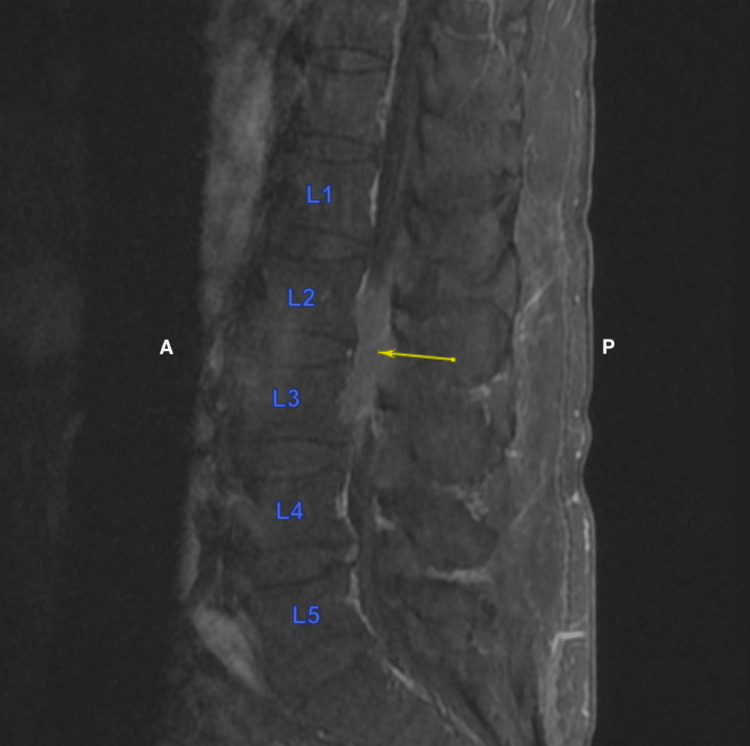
Extension of soft tissue in the vertebral canal and invasion into the vertebral body of L3 shown in the T1-weighted sagittal view with contrast A = Anterior; P = Posterior
